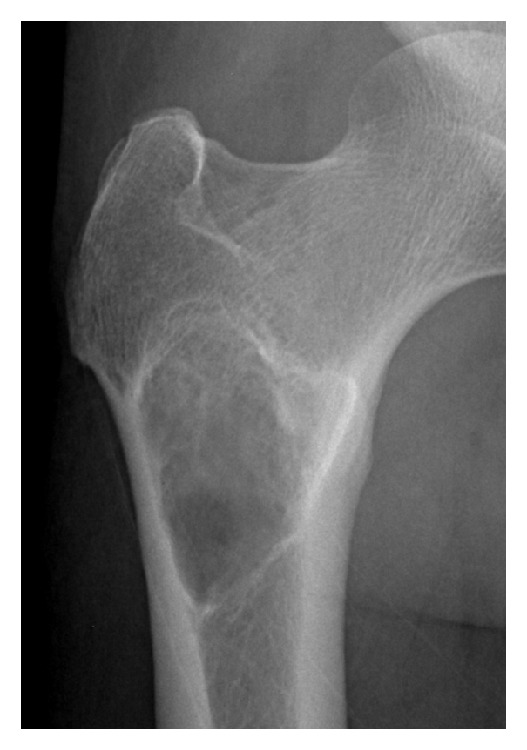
Images obtained from a 48-year-old male. Lesions in the femur demonstrated expansive growth, with internal ground glass opacities, an unbroken sclerotic rim, and no cortical involvement on X-ray.
